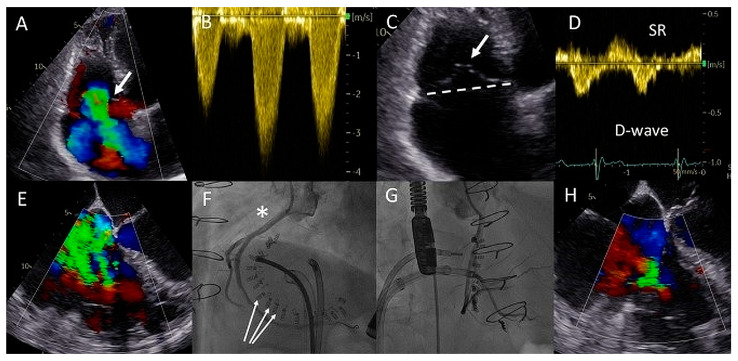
(A) Color Doppler apical four-chamber view shows torrential tricuspid regurgitation (TR) with a wide vena contracta (arrow) and flow disturbance filling the enlarged right atrium. (B) Continuous wave doppler showing a dense TR signal with elevated right ventricular pressures. (C) Noncoaptation of the tricuspid valve (TV) leaflets (arrow) and annulus dilatation (dash line, 53 mm) is seen. (D) The hepatic vein Doppler demonstrates a pattern in atrial fibrillation with a prominent and late peaking systolic reversal (SR) wave. The only forward flow is evident in diastole (D-wave). (E) Two TR jets (a vena contracta of 4 and 7 mm, respectively, ERO of 90 mm^2^, regurgitant volume of 98 mL) are evident during transesophageal imaging. (F) Left anterior oblique and (G) right superior oblique fluoroscopic views showing patent right coronary artery (asterisk) and 18 anchors (arrows) between the two TV commissures allowing the Cardioband to significantly reduce the annulus dimensions. (H) Transesophageal echocardiography showing significant reduction in TR after the Cardioband annulus reduction procedure (appreciate the difference with panel (E), vena contracta of 3 and 5 mm, respectively, ERO 35 mm^2^, regurgitant volume of 41 mL).
